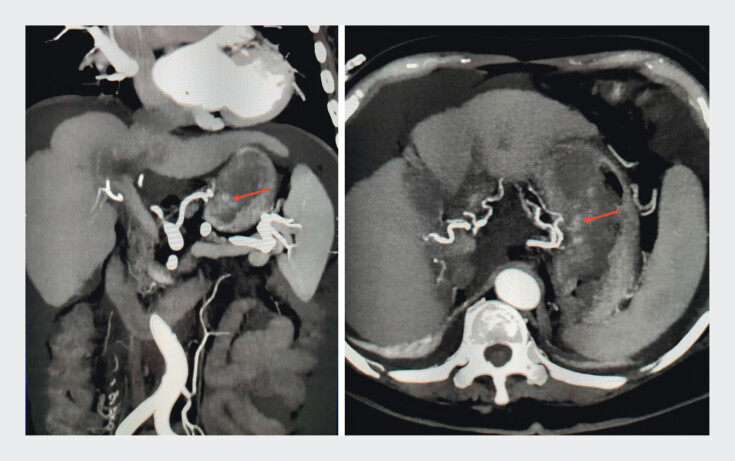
Contrast-enhanced computed tomography (CT) images before endoscopy: gastric wall thickening, uneven and slightly higher density in the lumen, marked enhancement of the lesion on the side of the lesser curvature of the stomach, and abundant blood supply.
